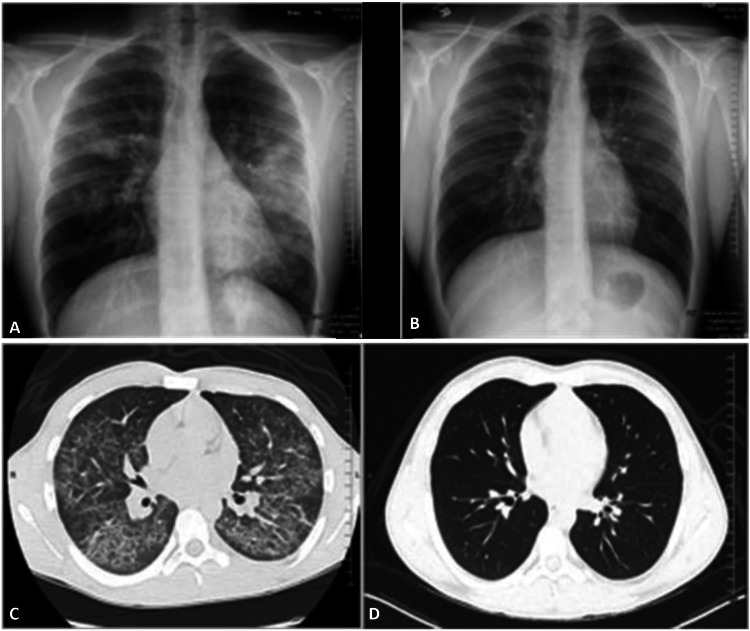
Chest Images A) Chest radiograph showing multifocal opacities, more on the left, including consolidation in the lingula, left lower lobe opacity, and right mid-lung field. B) Follow-up chest radiograph showing interval resolution of the previously noted bilateral air space opacities and mediastinal air. C) Chest computed tomography scan showing patchy areas of ground-glass opacification and interlobular septal thickening throughout both lungs with a lower lobe predominance and relative sparing of the periphery. D) Follow-up chest computed tomography scan showing near-complete resolution of the diffuse ground-glass opacification and interlobular septal thickening note on the prior study.
